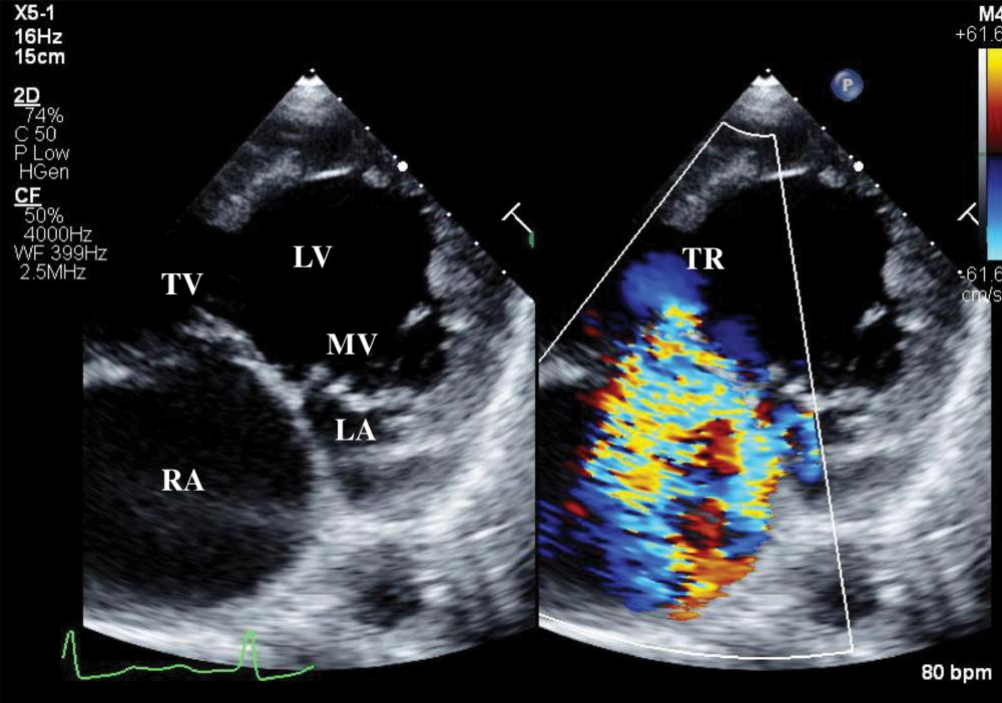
Baseline transthoracic echocardiography revealed a univentricular heart (double-inlet left ventricle) with severe tricuspid regurgitation. LV, left ventricle; TV, tricuspid valve; MV, mitral valve; RA, right atrium; LA, left atrium; TR, tricuspid regurgitation.
